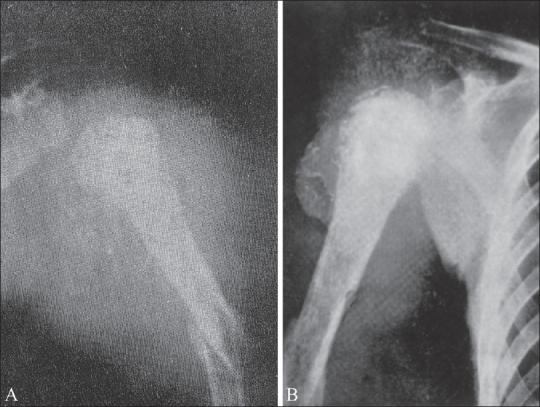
(A, B): Ewing's sarcoma of the humerus. Pre-irradiation radiograph (A), shows a lesion with marked condensation of bone with a large soft tissue swelling. Post-irradiation (B) radiograph shows regression of the soft tissue and bone condensation
